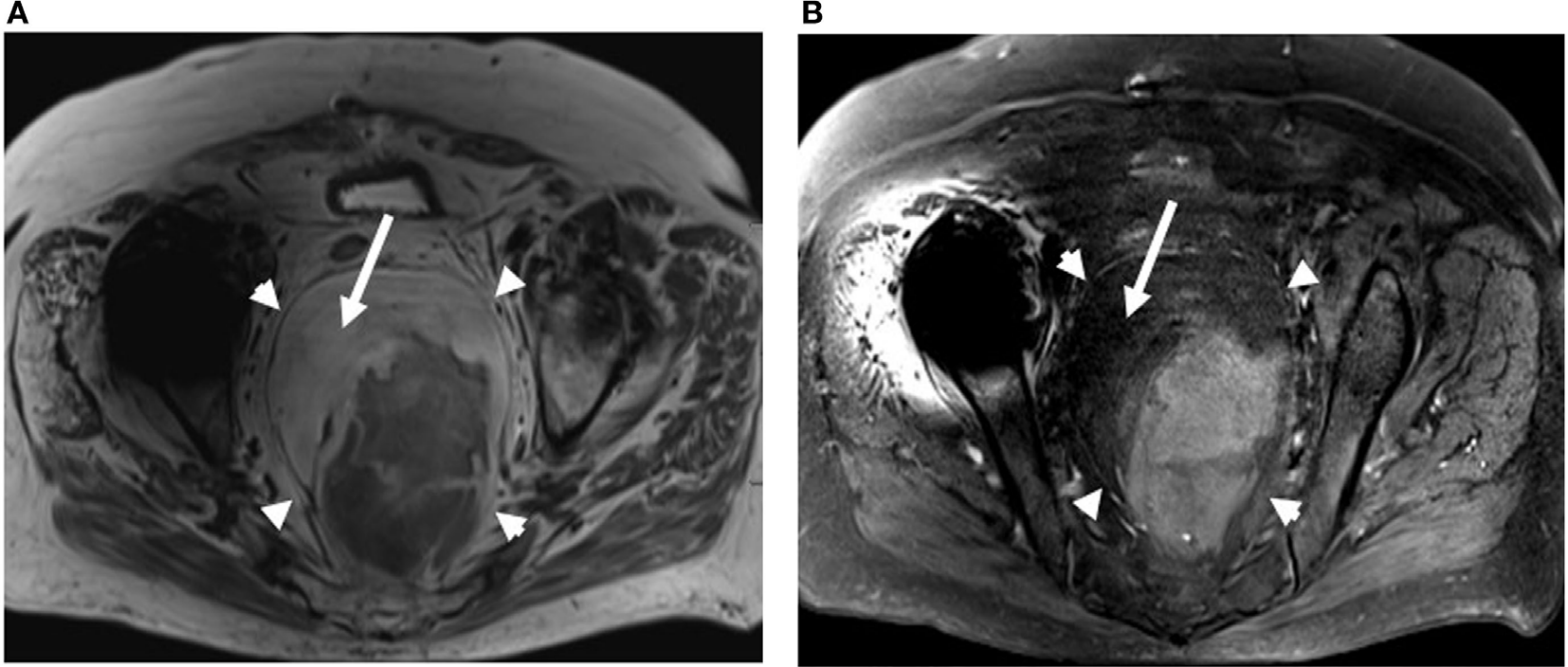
Axial nonfat saturated (A) and fat saturated (B) T2-weighted MRI images of the pelvis also demonstrates a large fat containing mass within the presacral region (arrowheads). Bright fat on nonfat saturated image (A) saturates out on the fat saturated image (B) (arrows).
